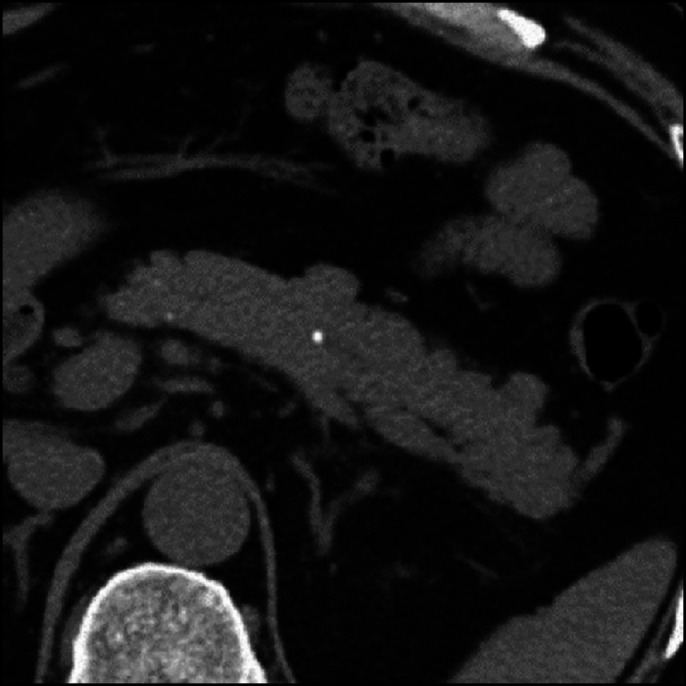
Small pancreatic calculus on computed tomography.
